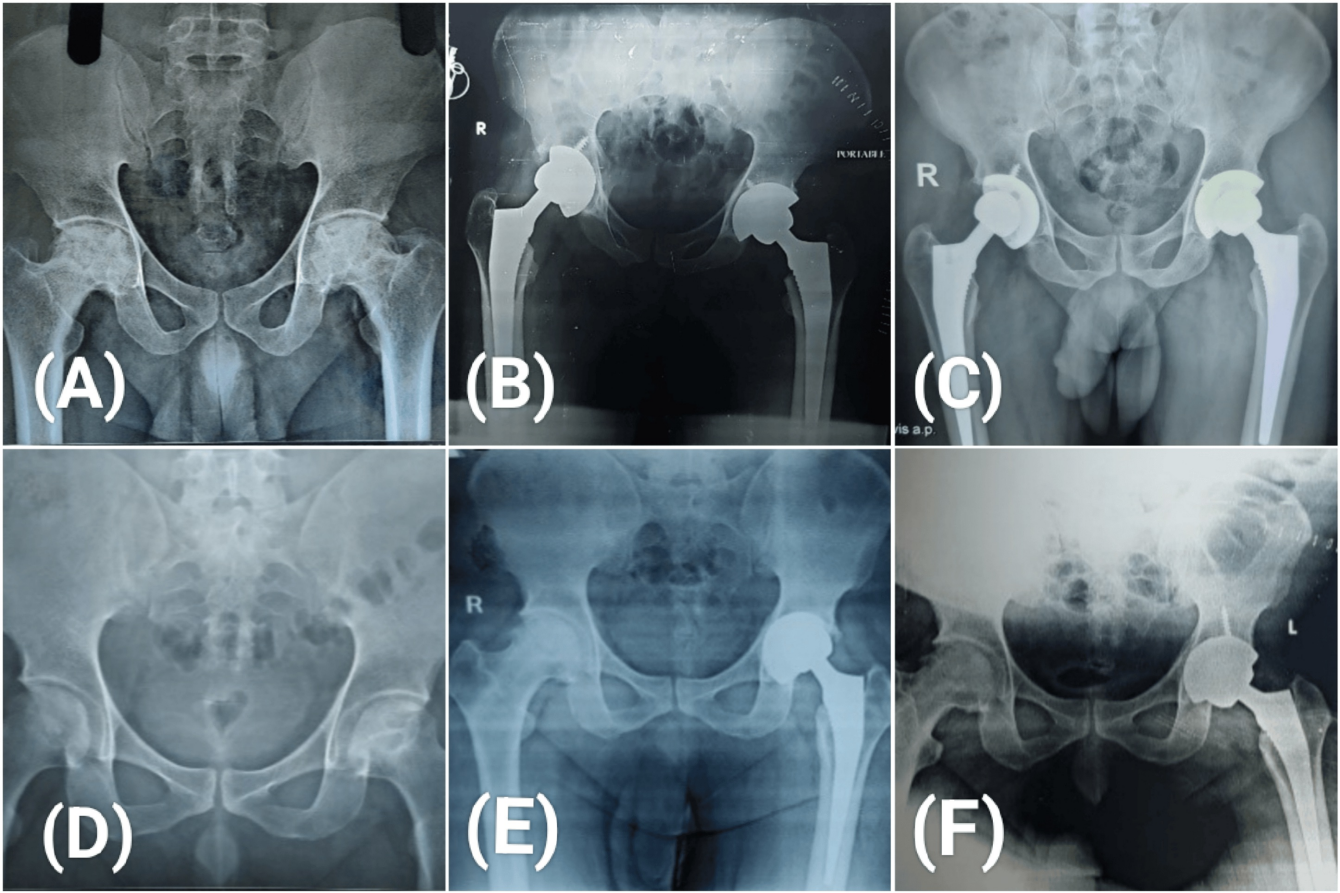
Patient 1- (A) Bilateral Stage Ⅲ ONFH; (B) Post-operative radiograph showing well-placed femoral and acetabular components over left side and high cup abduction angle in the right side; (C) Five-year follow-up showing well-fixed implants with good functional outcome and no reported complication. Patient 2- (D) Bilateral ONFH, Stage ⅡA right side and Stage Ⅲ left side underwent uncemented THA for left side and core decompression for right side; (E) Five-year follow-up radiograph showing low acetabular inclination angle and osteolysis (patient complained of squeaking and instability); (F) Post revision radiograph ONFH: Osteonecrosis of femoral head; THA: Total hip arthroplasty
